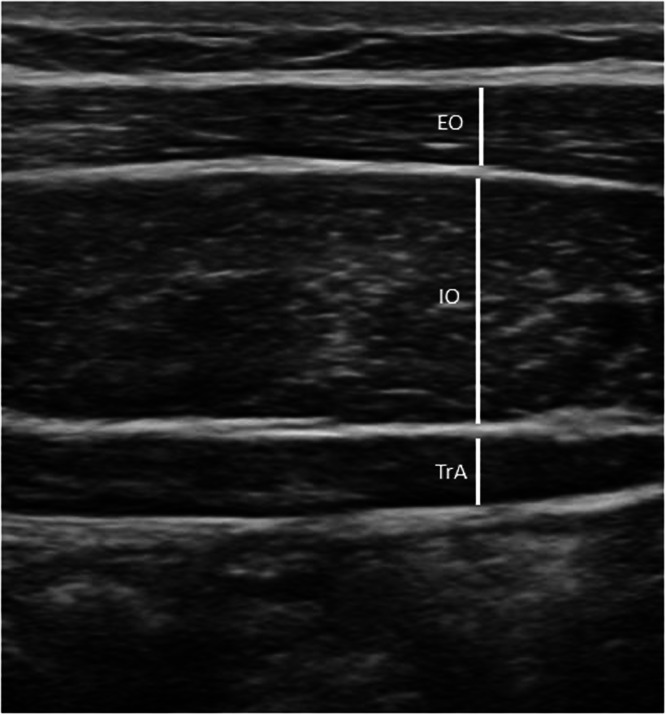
Ultrasound imaging of the deep abdominal muscles during assessment, demonstrating the transversus abdominis, internal oblique, and external oblique layers.
